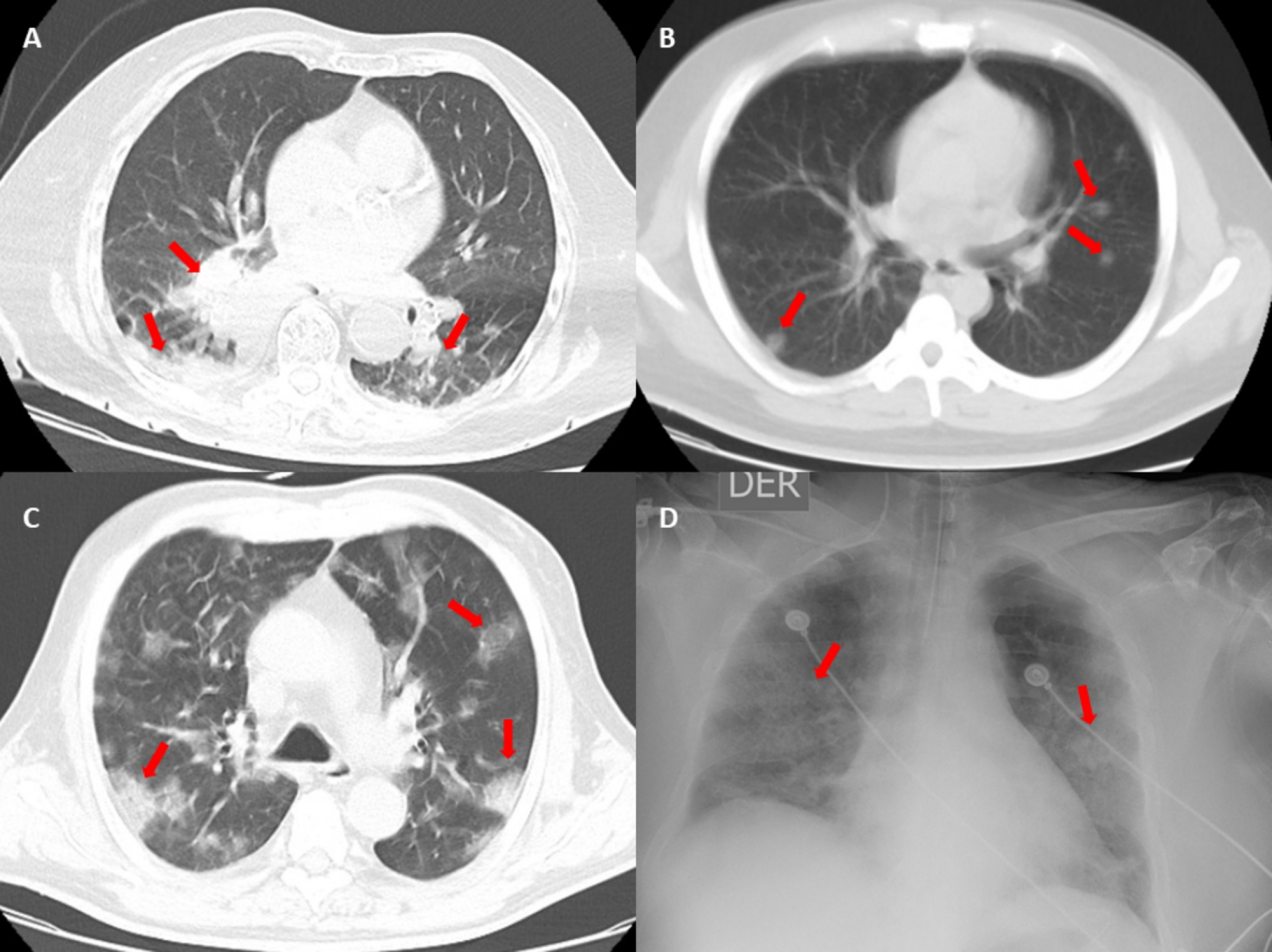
A: Chest CT at admission from Case 1. A mass that infiltrates the bronchi for the basal segments of the right lower lobe, as well as the presence of right basal pleural effusion and ground-glass consolidation. B: Chest CT at admission from Case 2. Multiple bilateral ground-glass opacities. C: Chest CT at admission from Case 3. Multiple bilateral crazy-paving consolidations. D: Chest radiograph at admission from Case 4. Multiple bilateral pulmonary consolidations.
